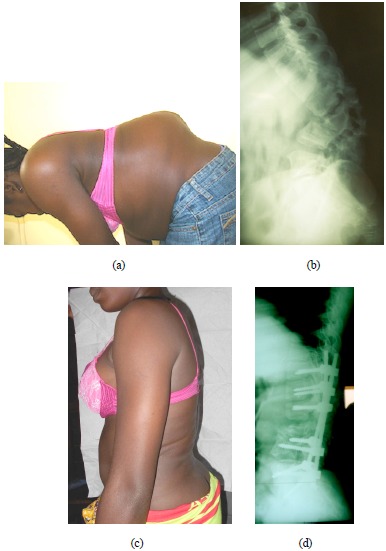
(a-b). Severe angular lumbar kyphosis involving destruction of L1, L2 and L3. Preoperative x-ray showing a 75° kyphosis. (c-d). Postoperative clinical and radiographic aspect after correction by pedicle substraction osteotomy and instrumentation from T11 to S1.
